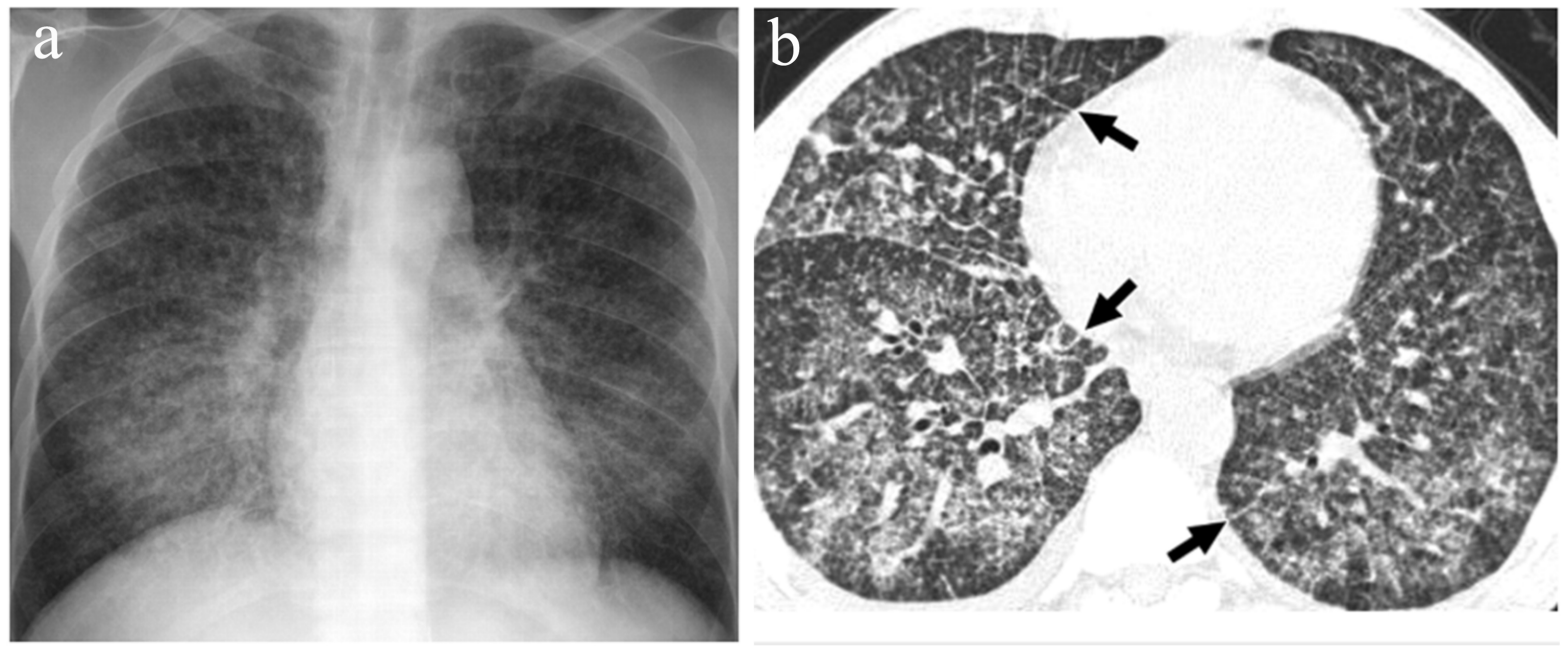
Imaging findings of tracheobronchial tuberculosis. (a) Chest X-ray for a man suffering from the tracheobronchial form of tuberculosis. Chest X-rays of patients with tracheobronchial tuberculosis may show only subtle changes; “definite” airway lesions are absent, therefore, yielding a low diagnostic sensitivity of routine X-rays. (b) High-resolution CT scan demonstrates small nodules and “tree bud signs” most appropriately seen within the lung parenchyma, bronchiogenic spread. These changes are often difficult to demonstrate with conventional X-rays. With HRCT, the intrinsic nature, scope and severity of lesions have the accuracy which is significant for the early diagnosis of TBTB.
